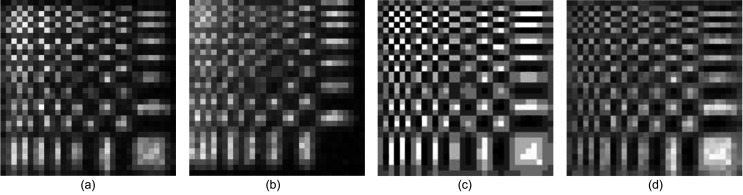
Pinhole images of the 32 × 32 quaternary mask. (*a*) Mean X-ray bucket image obtained for the *P* mask. (*b*) Mean bucket image for the *N* mask. (*c*) Original Huffman-like array, black = −3, white = +3. (*d*) Reconstructed X-ray pinhole image of the 32 × 32 Huffman-like mask.
